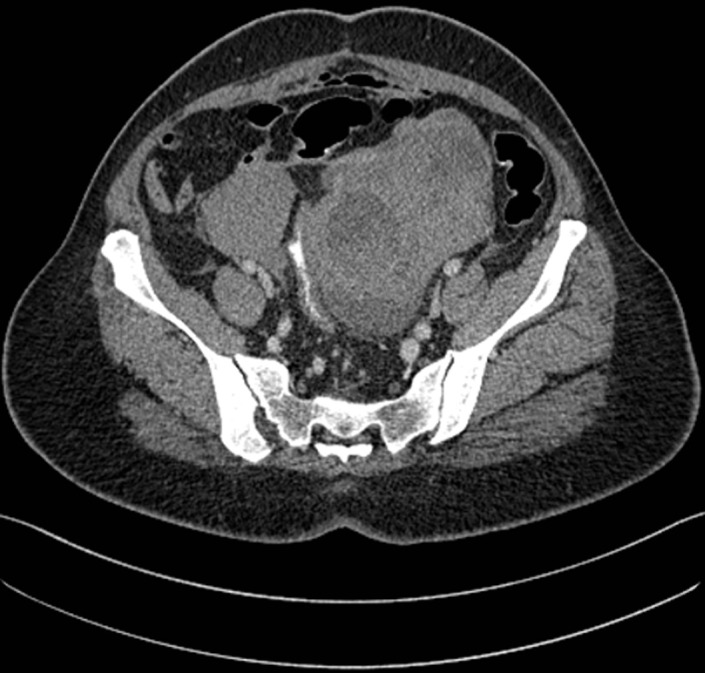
computerized tomography (CT) of pelvis showing bilateral enlarged irregular heterogeneously enhancing masses; several cystic components seen in the mass suggestive of necrosis; no contrast extravasation suggestive of active bleeding is seen
